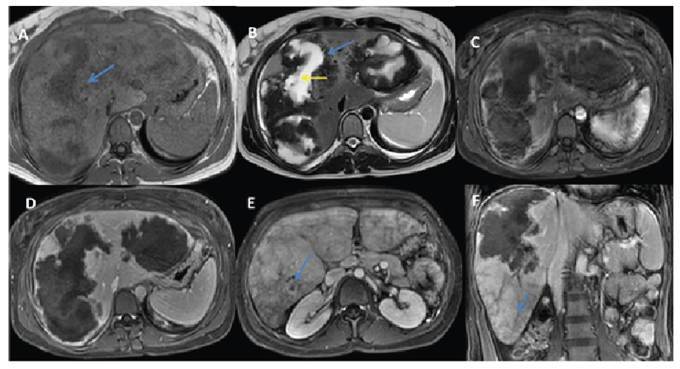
Magnetic resonance imaging findings. (A) T1-weighted images show hypointense lesions (blue arrow), while (B) T2-weighted images demonstrate a hypointense peripheral rim (blue arrow) with central hyperintensity (yellow arrow). Mild peripheral contrast enhancement is visible in the arterial phase (C), becoming more pronounced in the delayed phases (D). Perfusion reduction secondary to portal vein thrombosis (E, blue arrow), along with a heterogeneous parenchymal appearance (F, blue arrow).
